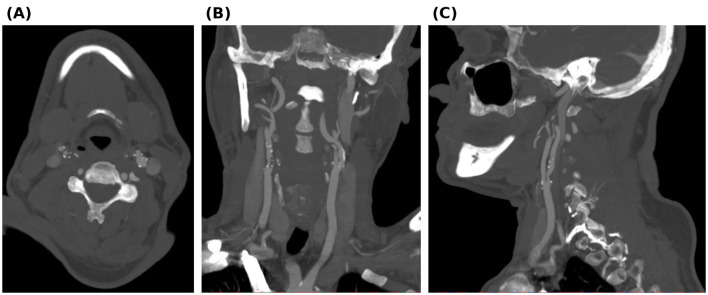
Arterial-phase computed tomography angiography (CTA) of the same patient as in Figure 1, demonstrating 70%−99% stenosis (NASCET) of the right internal carotid artery (RICA). (A) Axial reconstruction at the level of the cervical spine. (B) Coronal maximum-intensity projection (MIP) of the cervical carotid arteries. (C) Sagittal MIP of the right cervical carotid system. Multiplanar CTA reconstruction provides accurate quantification of stenosis severity, plaque morphology, calcification, and lesion length, parameters that are critical for procedural planning. Source: authors' personal archive.
